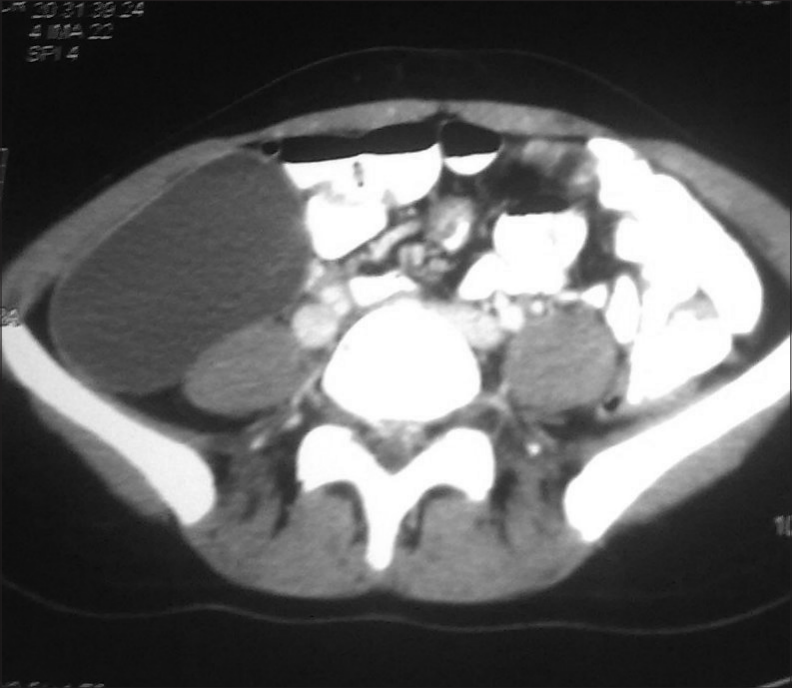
Contrast enhanced CT scan showing unilocular swelling arising from psoas muscle cystic mass in dimensions measuring 19.4×11×11.6 cm^3^ suggestive of hydatid cyst
